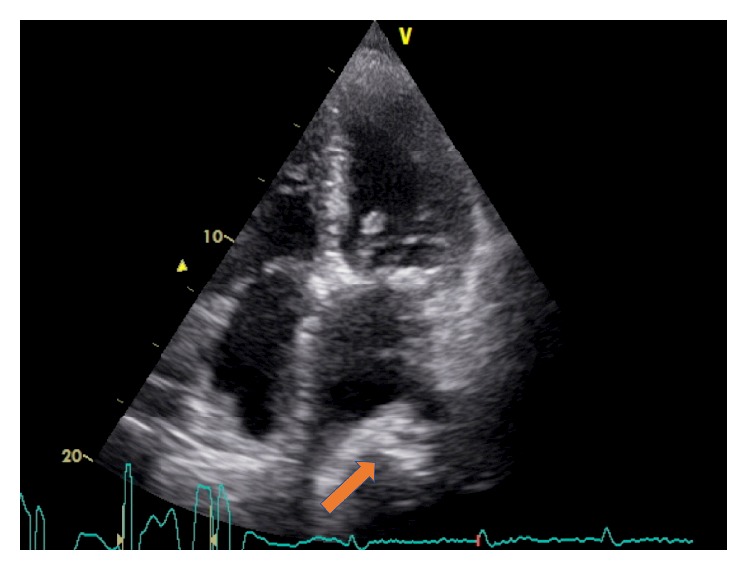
Echocardiography (four-chamber view) of the heart depicting a mass compressing the left atrium.
